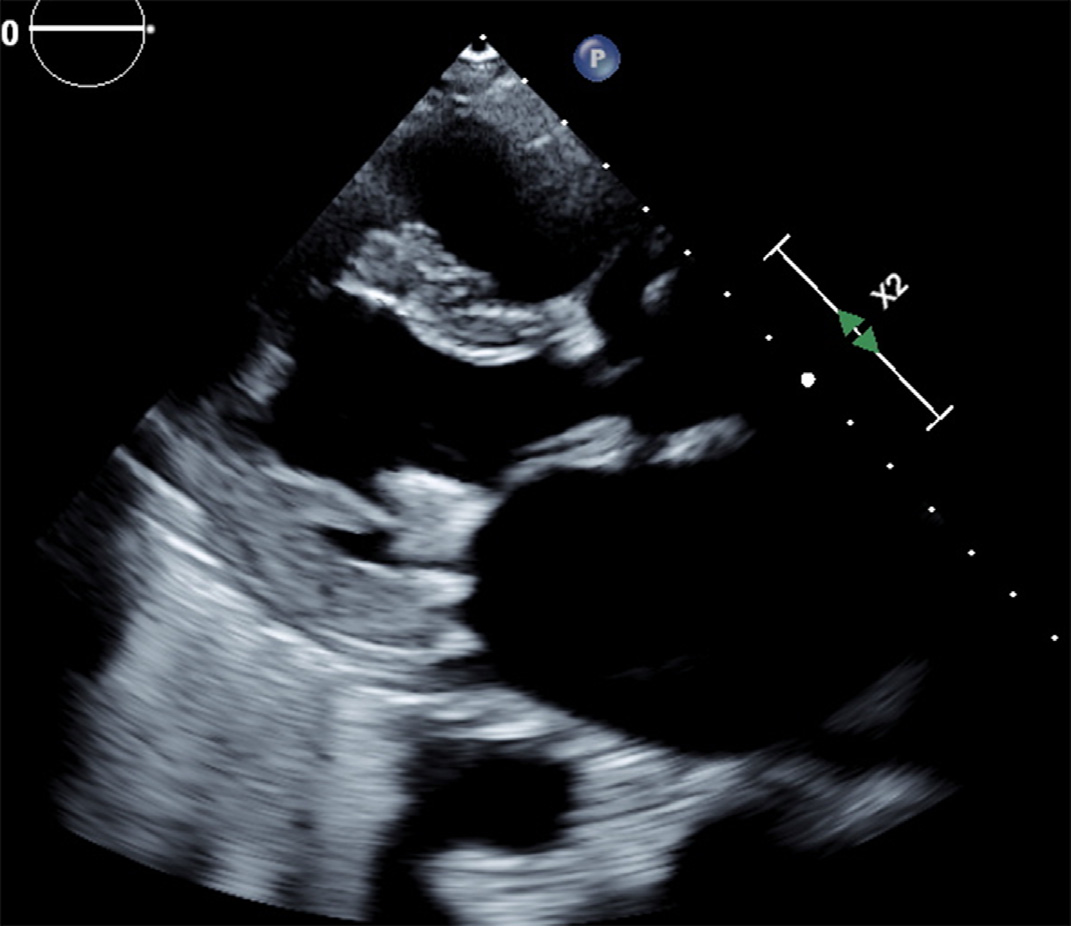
Transthoracic Echocardiogram Parasternal long-axis view demonstranting no pericadial effusion.
